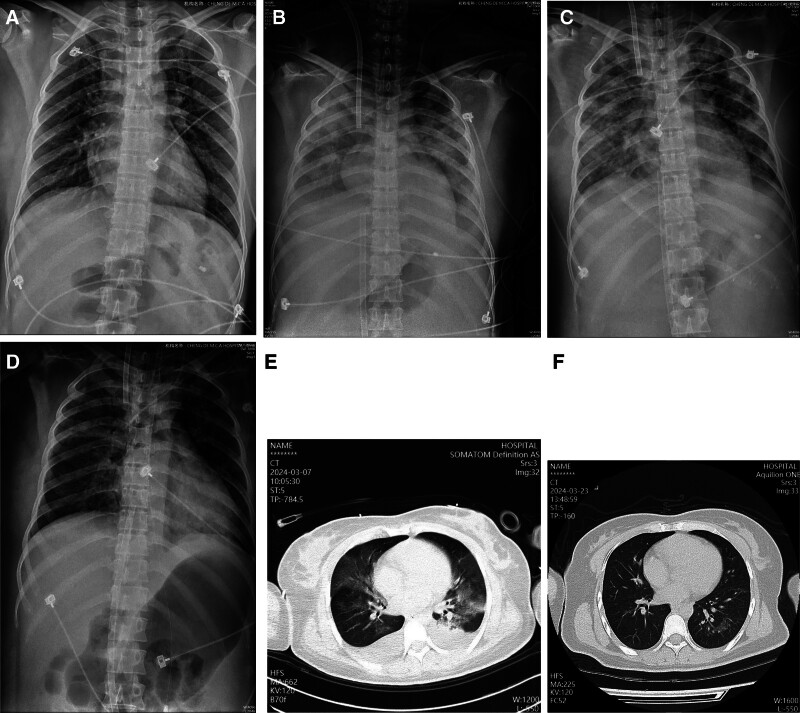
Imaging presentation, transformation, and outcome of the patient. (A) Bedside chest radiography on the day of admission, (B) after 4 hours of VA-ECMO treatment, (C) after VAV-ECMO treatment, (D) bedside chest radiography before ventilator withdrawal, (E) lung CT scan after withdrawal of the ventilator, and (F) lung CT scan before discharge. ECMO = extracorporeal membrane oxygenation, VA-ECMO = veno-arterial ECMO, VAV-ECMO = veno-arterial-venous ECMO.
